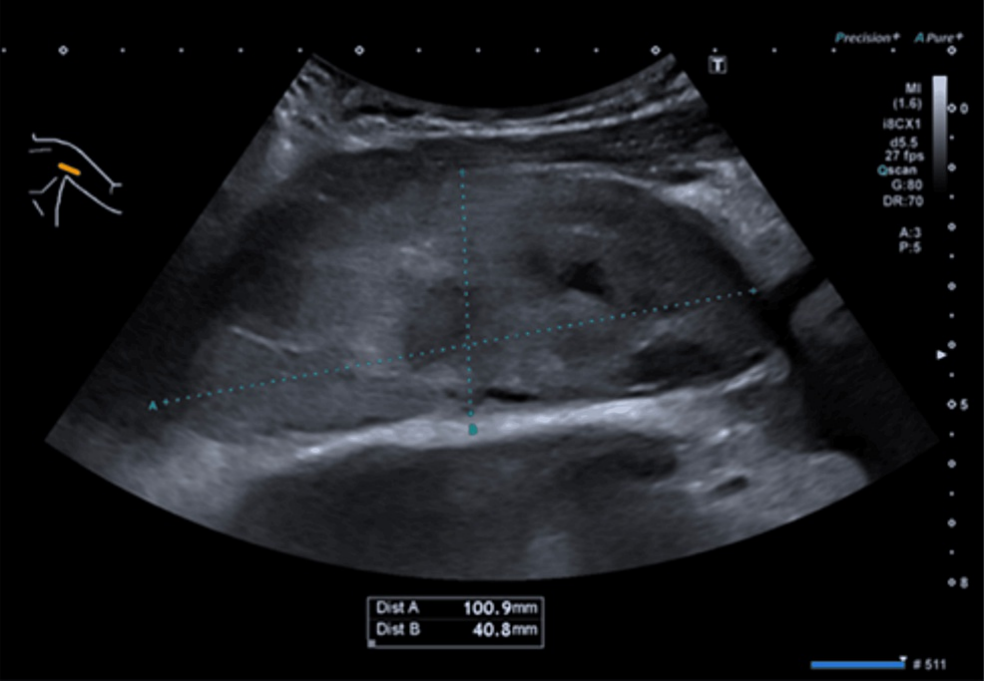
Ultrasound scan of the haematoma in the left axilla. Ultrasound scan showing a large well-defined area of mixed echogenicity measuring 10 x 5 cm (longitudinal x AP). In keeping with an intramuscular haematoma of the left axilla.
